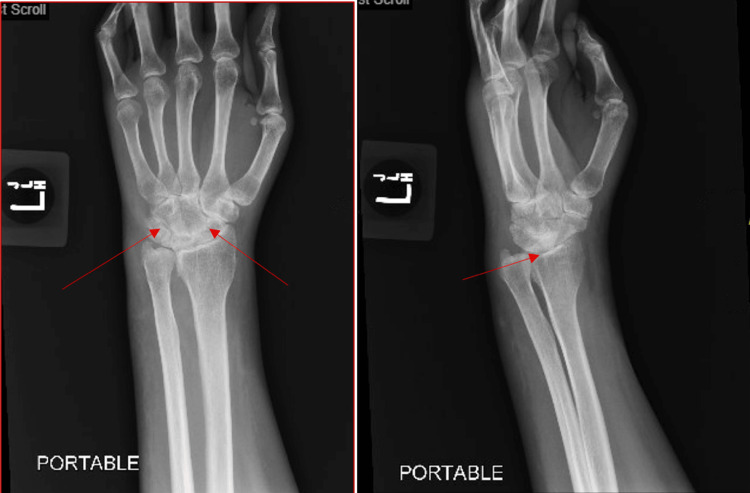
Radiograph of the left wrist demonstrating arthropathic changes with joint space narrowing involving the carpal articulations. Chondrocalcinosis of the radiocarpal joint is present, along with mild diffuse soft tissue swelling.
